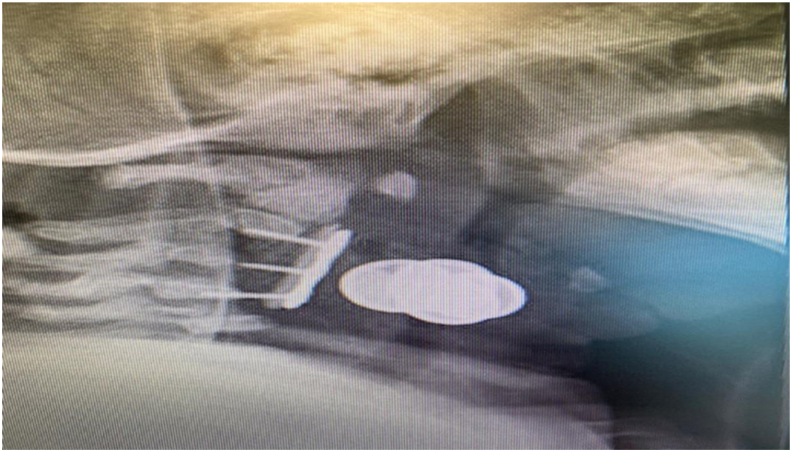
A cervical spine x-ray was done 4 months after follow-up showing properly placed plate and screws over the body of C2 iliac bone graft and C4.
